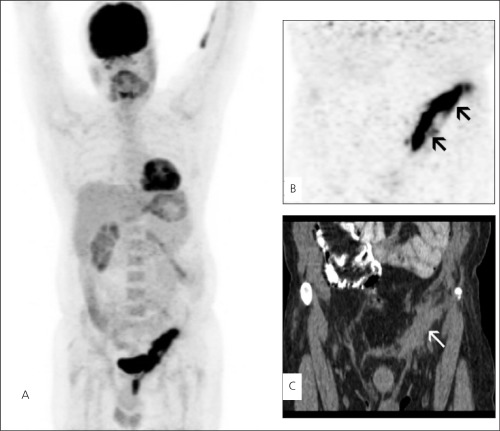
A, B, C. Maximum intensity projection (MIP) images (A) andcoronal PET image (B) show increased FDG uptake on left inguinalregion (arrows). Coronal CT image (C) demonstrates soft tissue thickening(white arrow)
